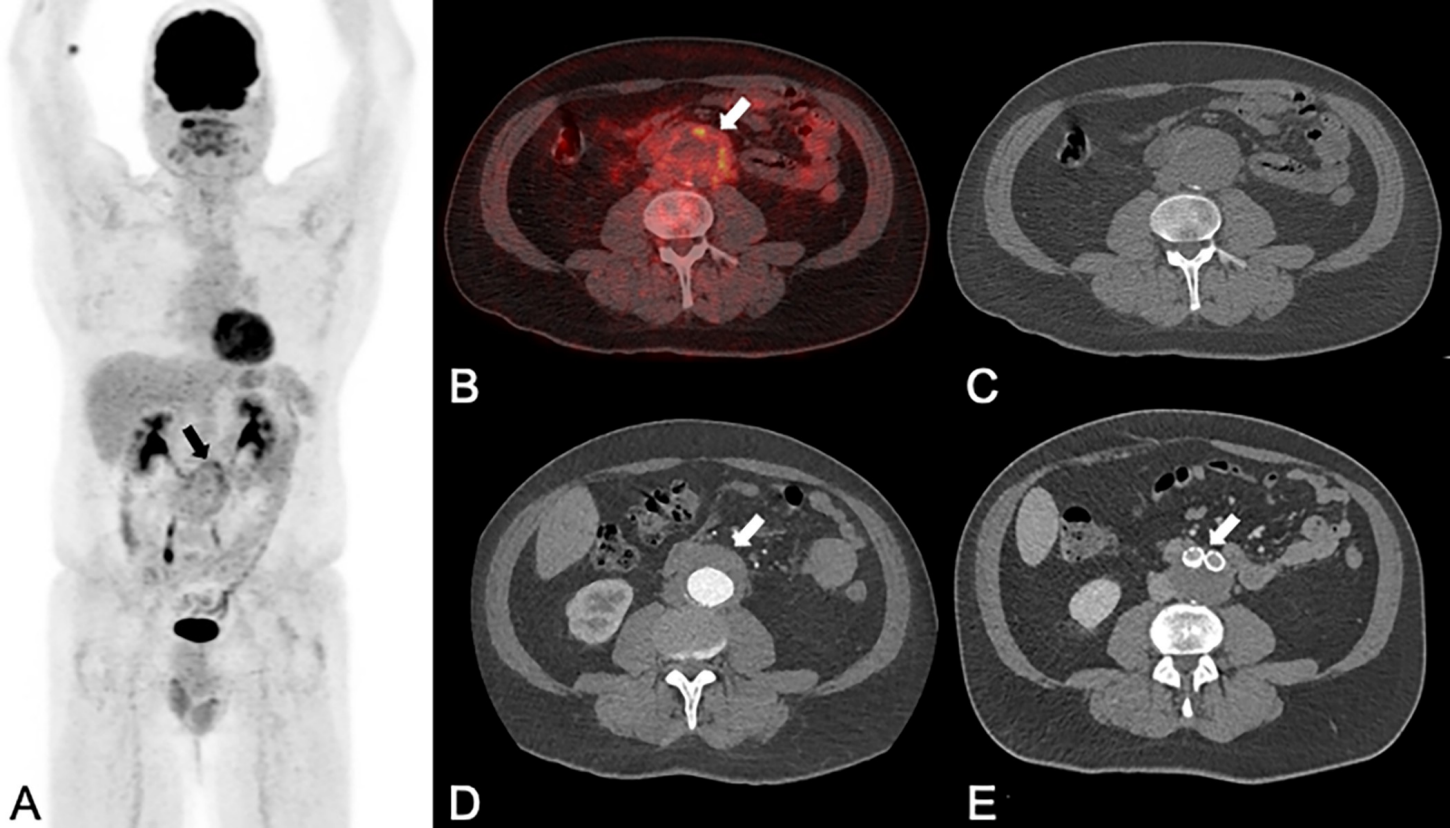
A 48-year-old man with a non-infected, non-inflammatory abdominal aortic aneurysm, initially diagnosed as an incidental finding on abdominal ultrasound. PET/CT (A: maximum intensity projection of PET; B: fused PET/CT images (B); C native CT images) and contrast-enhanced CT (CE-CT: D) showed a 55 mm large abdominal aortic aneurysm (white arrows in B and D) with increased FDG uptake (SUVmax 5.2, black arrow in A, white arrow in B) in the thickened (20 mm) aortic wall (white arrow in D). Both readers rated all imaging data set falsely: using PET/CT images and CE-CT images alone, one reader diagnosed a mycotic/infectious aneurysm while the other one diagnosed an inflammatory aneurysm; both readers suspected an inflammatory aneurysm on combined imaging. The patient was subsequently treated with percutaneous endovascular abdominal aortic aneurysm repair and neither antibiotic nor steroid therapy. The latest follow-up imaging four years after the initial diagnosis, showed no residual wall thickening abdominal aorta (E) and the patient had no abdominal symptoms.
